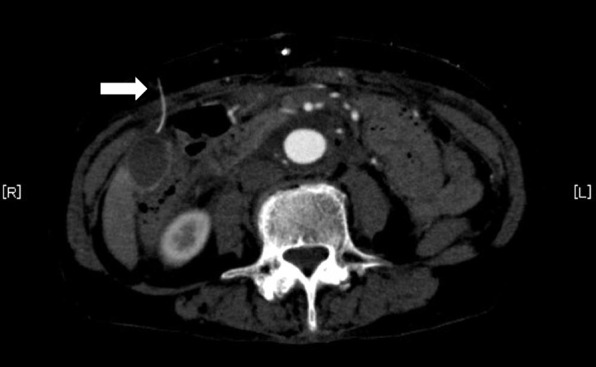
A computed tomography scan (transverse view) on Postoperative Day 1. The white arrow in the figure indicates the catheter, which strayed into the abdominal cavity
